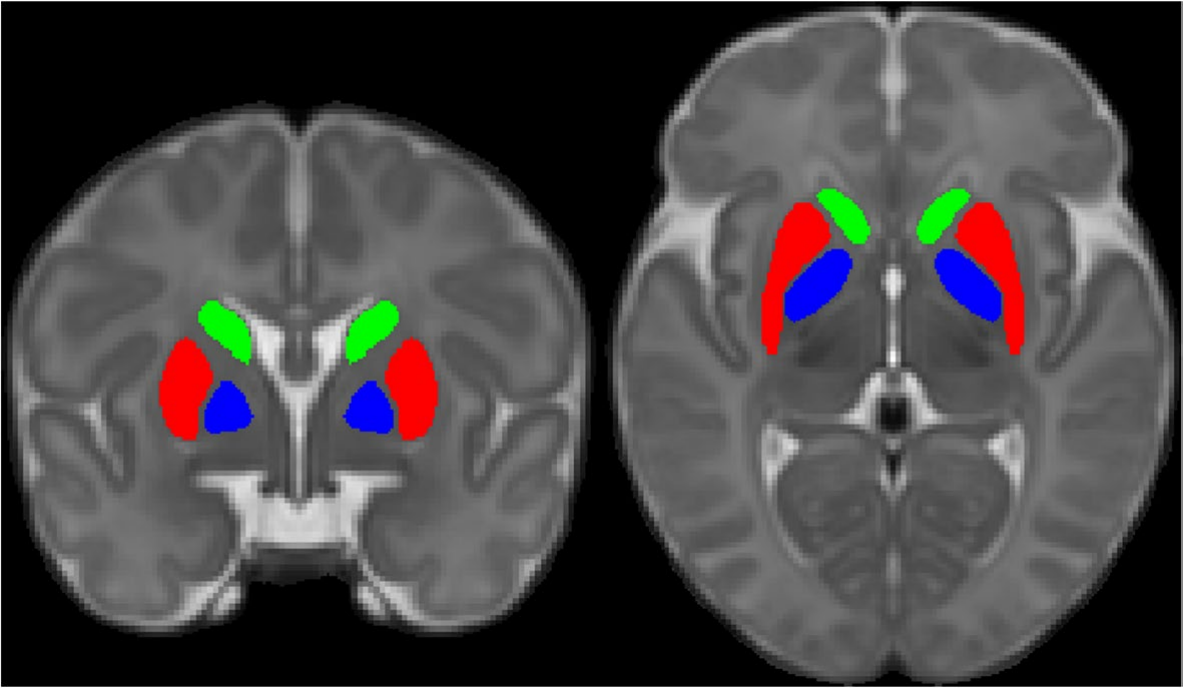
The bilateral regions of interest used in the study; caudate nucleus (in green), putamen (in red), and globus pallidus (in blue) are presented on a T2-weighted sample average brain image
